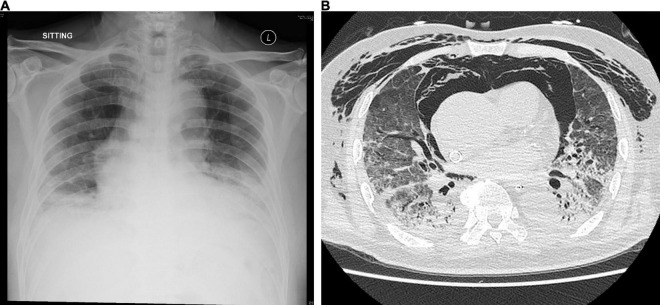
(A) Chest radiograph on admission showing bilateral lower zone reticulonodular shadows and (B) CT scan of the thorax on day 15 showing mixed consolidative and atelectatic changes in the dependent regions of both lungs.
